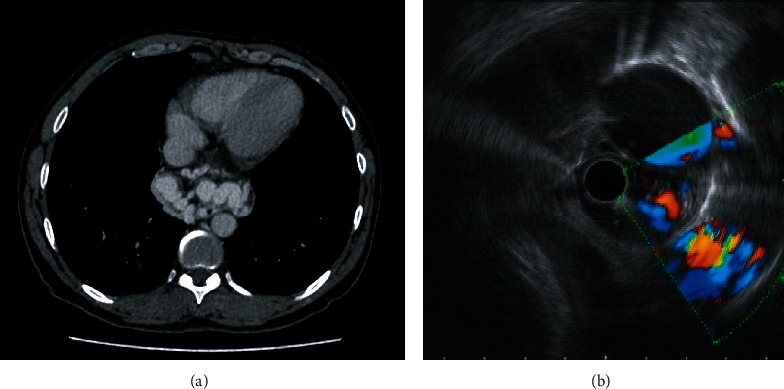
CT and endoscopic ultrasonography(EUS) findings of extraluminal collaterals. (a) Large paraesophageal veins on CT (patient #1). (b) Large paragastric veins on EUS (patient #5).
